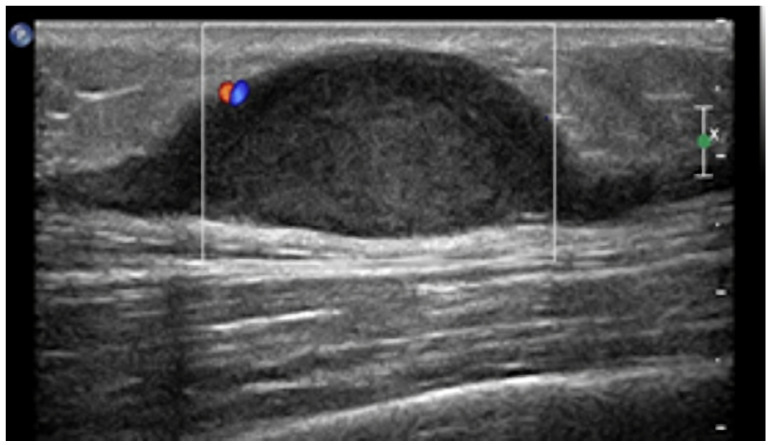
US appearance of schwannomas. Most schwannomas arise from the nerve sheath of peripheral nerves and occur below or at the level of the subcutaneous fat layer and, as shown, appear as hypoechoic fusiform mass with small blood flow on color Doppler and, sometimes, peripheral nerve continuity.
